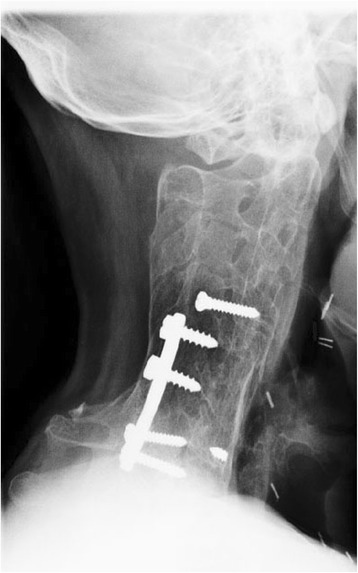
Lateral 24 year follow up radiographs of the cervical spine of the patient
